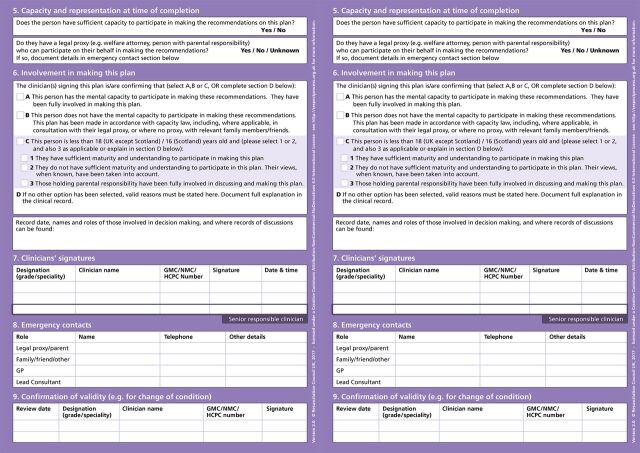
ReSPECT form, V.2.0. CPR, cardiopulmonary resuscitation; GP, general practitioner; NHS, National Health Service; ReSPECT, Recommended Summary Plan for Emergency Care and Treatment.
